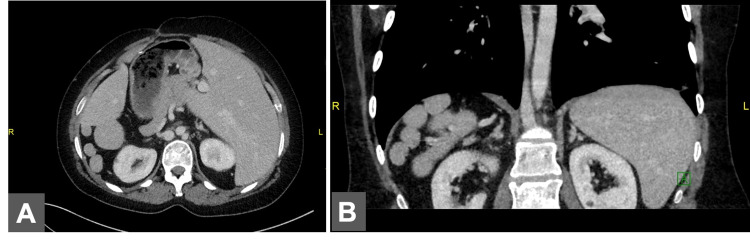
Axial and coronal abdominal CT images A. Axial abdominal CT image. B. Coronal abdominal CT image demonstrating polysplenia CT: computed tomography
